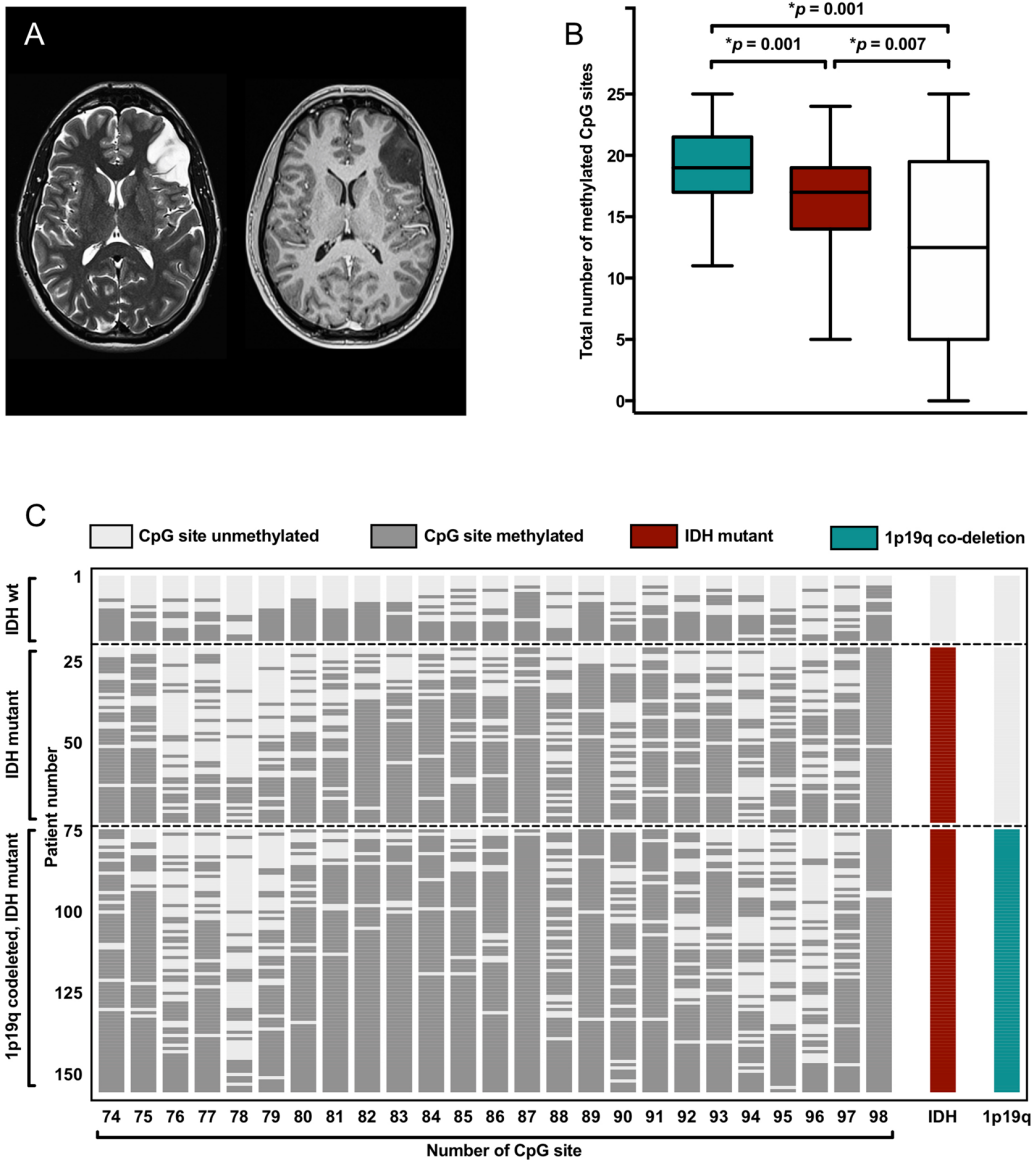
Extent and pattern of MGMT promotor methylation. (A): Axial brain MRI with T2-weighted (left panel) and T1-weighted post-contrast (right panel) sequences shows diffuse astrocytoma without contrast-enhancement. (B) Number of methylated CpG sites in patients with 1p19q co-deleted oligodendroglioma (cyan), IDH mutant astrocytoma (red), and IDH wild-type astrocytoma (white). Median, interquartile range, and total range are given. (C) Methylation pattern of CpG sites 74–98 within the MGMT promotor region in 155 patients with glioma WHO grade II. Each row corresponds to an individual patient, and each column to a different CpG site. Dark grey rectangles represent methylated sites and light grey rectangles represent unmethylated sites. IDH and 1p19q status are also indicated for each patients and color-coded.
